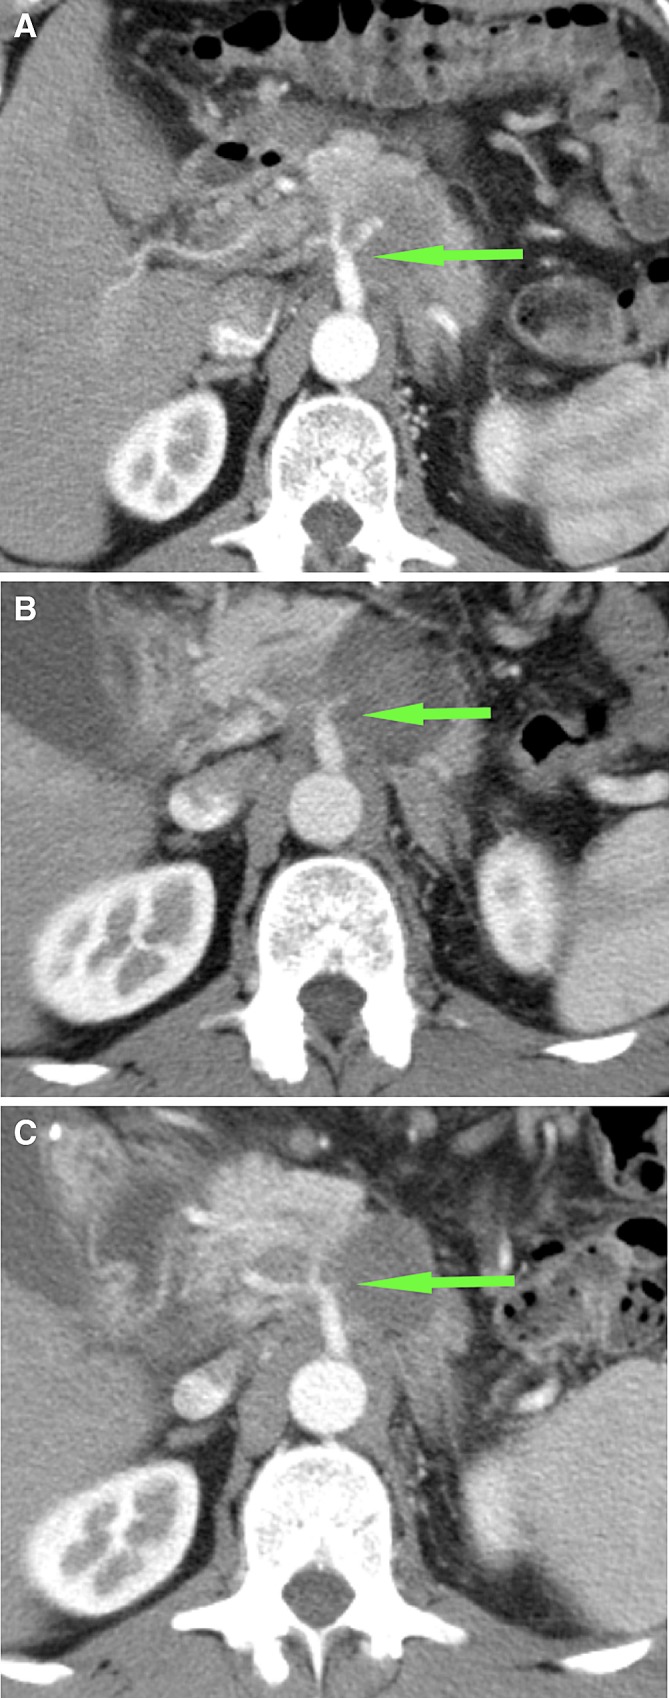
CT-images in the axial plane, of a 51-year-old man with a ductal adenocarcinoma in the corpus of the pancreas. A On the CT-imaging before RFA, the coeliac trunk and hepatic artery are closely related to the tumor. B One week after the procedure, these arteries are constricted but still patent (arrow). C This remained stable during follow-up 3 months later (arrow)
